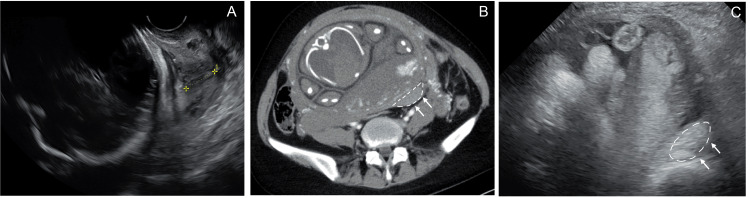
Ultrasonographic and computed tomographic findings of ruptured ovarian endometrioma during pregnancy. (A) Transvaginal ultrasonography revealed cervical shortening. (B) Transabdominal ultrasonography image showing a hematoma posterior to the uterus. The bleeding site is indicated by dotted lines and arrows. (C) Contrast-enhanced abdominal computed tomography image showing a high-density area posterior to the uterus, suggestive of a hematoma (indicated by dotted lines and arrows).
